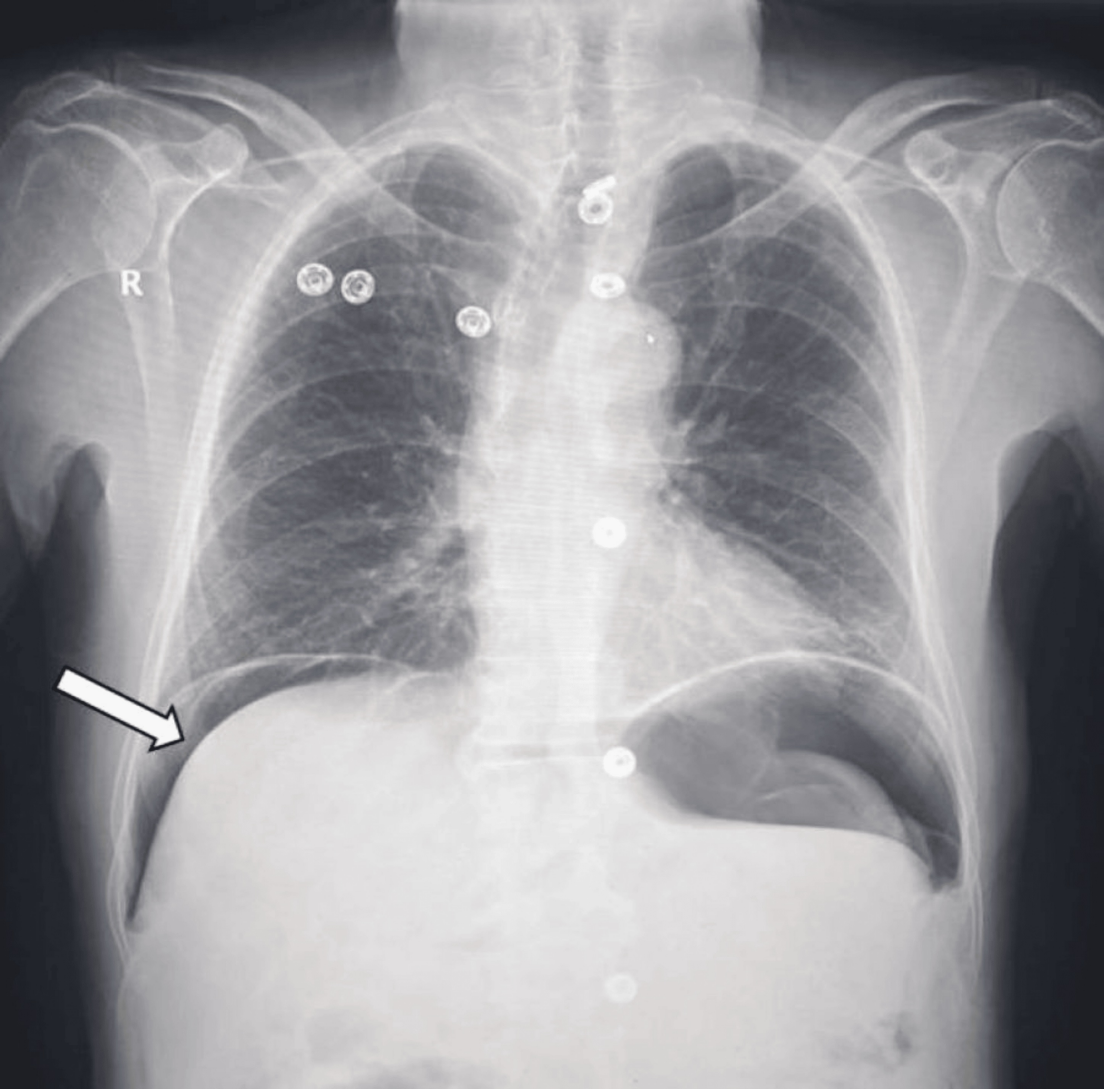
Erect abdominal X-ray showing air under diaphragm (pneumoperitoneum, arrow).
